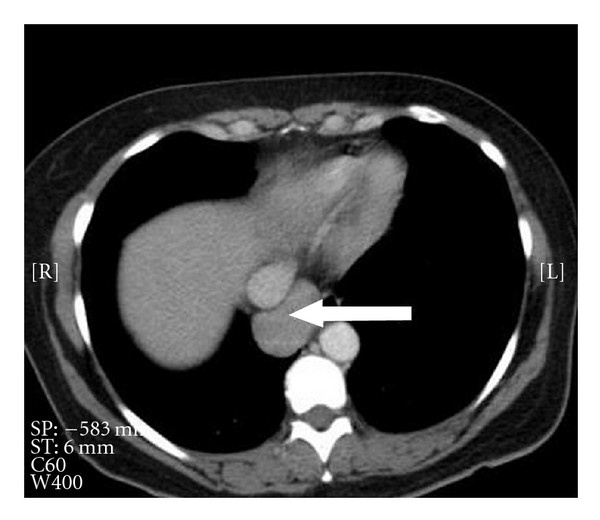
The CT scan showing a 4.5 × 3.3 cm mildly enhancing soft tissue mass in the right posterior mediastinum.
